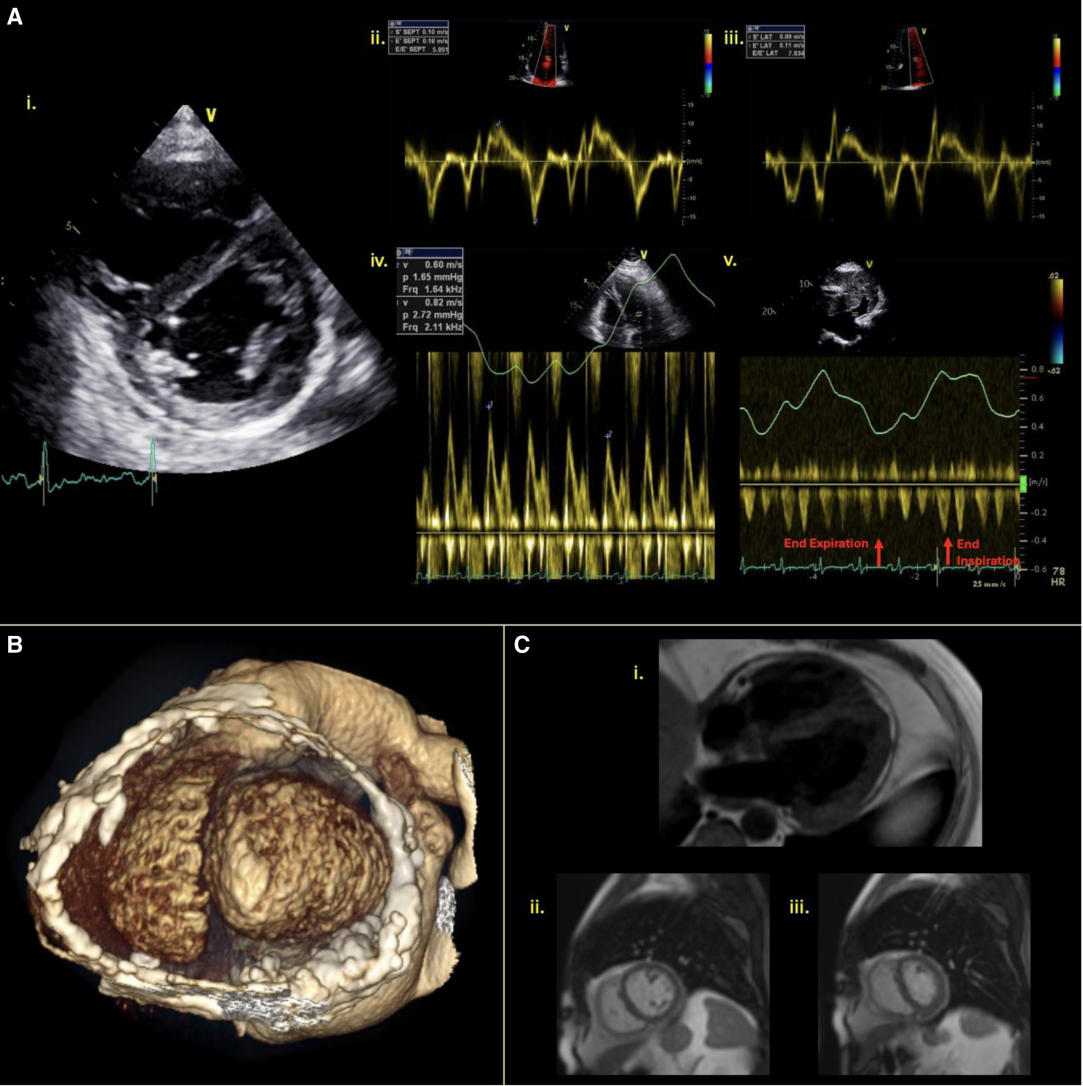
A 54-year-old man with pericardial constriction after chest radiotherapy for lymphoma aged 18 years. A, Transthoracic echocardiography images demonstrating pericardial thickening, increased echogenicity as well as septal flattening with inspiration (i), annulus reversus with e′ sept 0.16 m/s and e′ lateral 0.11 m/s (ii and iii) >25% respiratory variation of transmitral E-wave velocity (iv) and decreased hepatic vein forward flow with expiration (v). B, Three-dimensional computed tomography reconstruction showing extensive pericardial calcification. C, Cardiovascular magnetic resonance imaging showing pericardial thickening on T1 black-blood imaging (i) and deformation of left ventricular with inspiration (ii) with resolution with expiration (iii).
